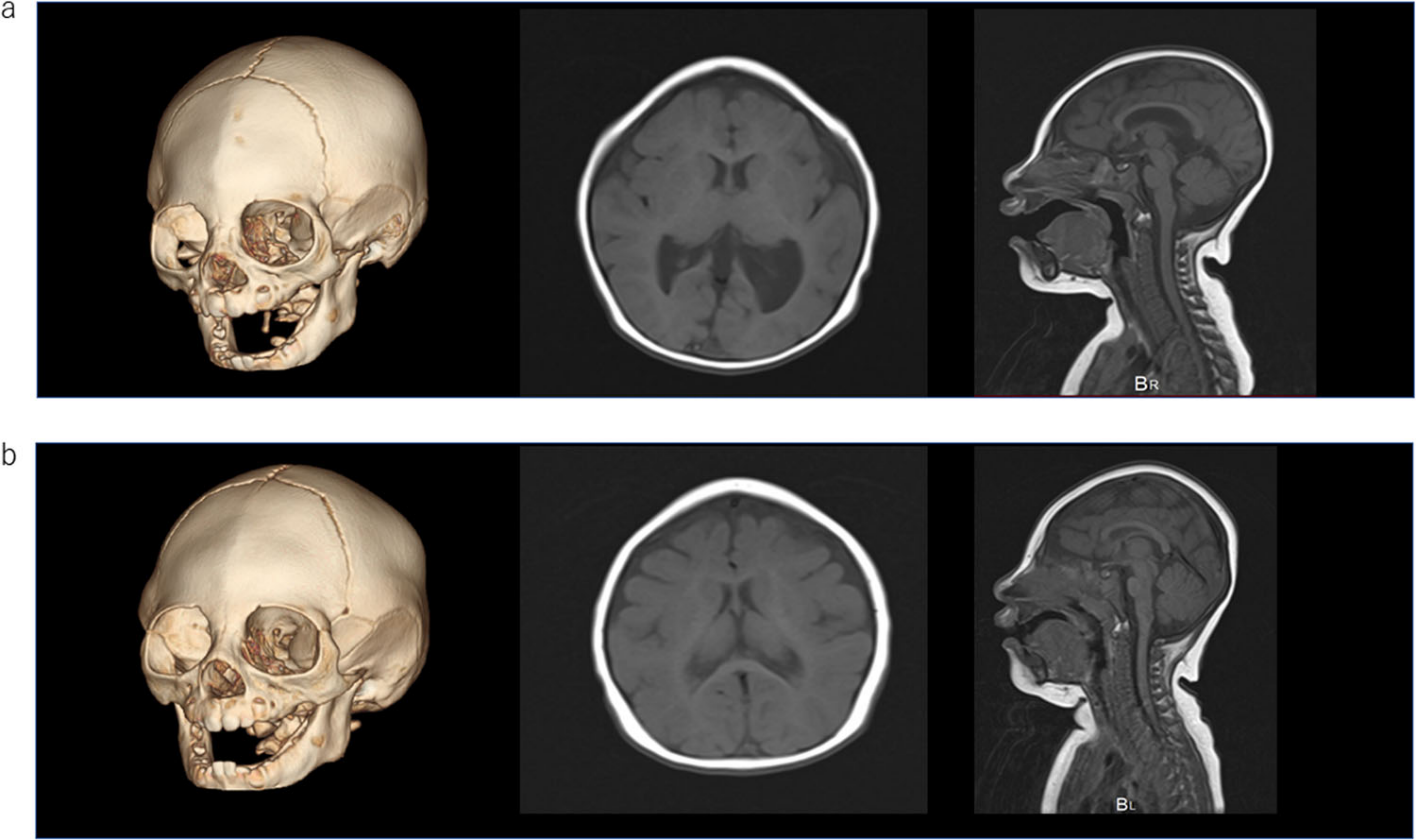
Brain imaging. a The older sister at 2 years of age. Three-dimensional CT (left), axial (middle), and sagittal (right) T1-weighted MRI. Metopic suture craniosynostosis led to trigonocephaly. Significant small frontal lobes, small pons, and enlarged lateral ventricles were observed. Cerebellar hypoplasia was not observed. b The younger brother at 6 months of age. The findings were similar to his sister’s, but he did not have lateral ventricle enlargement. Cerebellar hypoplasia was not observed, just as in his sister.
